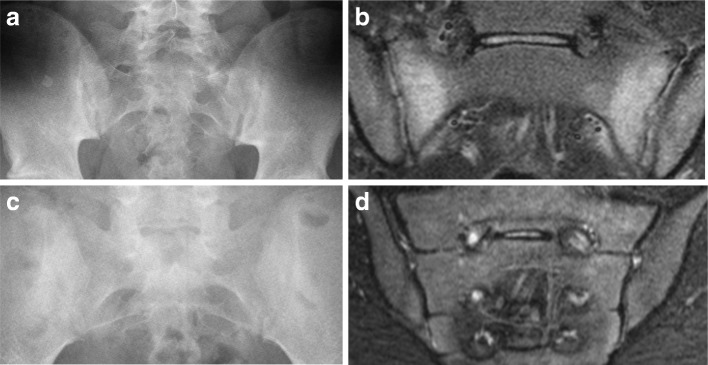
Samples of discordant radiograph and MRI overall impressions of presence/absence of sacroiliitis. a and b) 16-year-old HLA-B27+ male with 2 months of hamstring, gluteal, and low back pain. (a) Radiograph – normal (no sacroiliitis) by 5/5 raters; two raters noted sclerosis. (b) MRI STIR – abnormal (sacroiliitis present) rated by 5/5 raters; bilateral sacral subchondral bone marrow edema is clearly present. Two raters reported a positive erosion finding and 3 raters reported a positive sclerosis finding. (c and d) 13-year-old HLA-B27 negative female with lower and mid-back pain with accompanying morning stiffness, acute uveitis, and multiple tender entheses. (c) Radiograph – rated abnormal (sacroiliitis present) by 5/5 raters; two raters reported a positive finding for erosion, two reported a finding of joint space narrowing, and all five raters reported sclerosis. (d) MRI STIR – rated as normal (no sacroiliitis) by 5/5 raters; no abnormalities (erosion, sclerosis, fat metaplasia, ankylosis, or backfill) were reported on MRI. Radiologist raters were blinded to patient clinical details and are included here to provide the reader with relevant medical history
